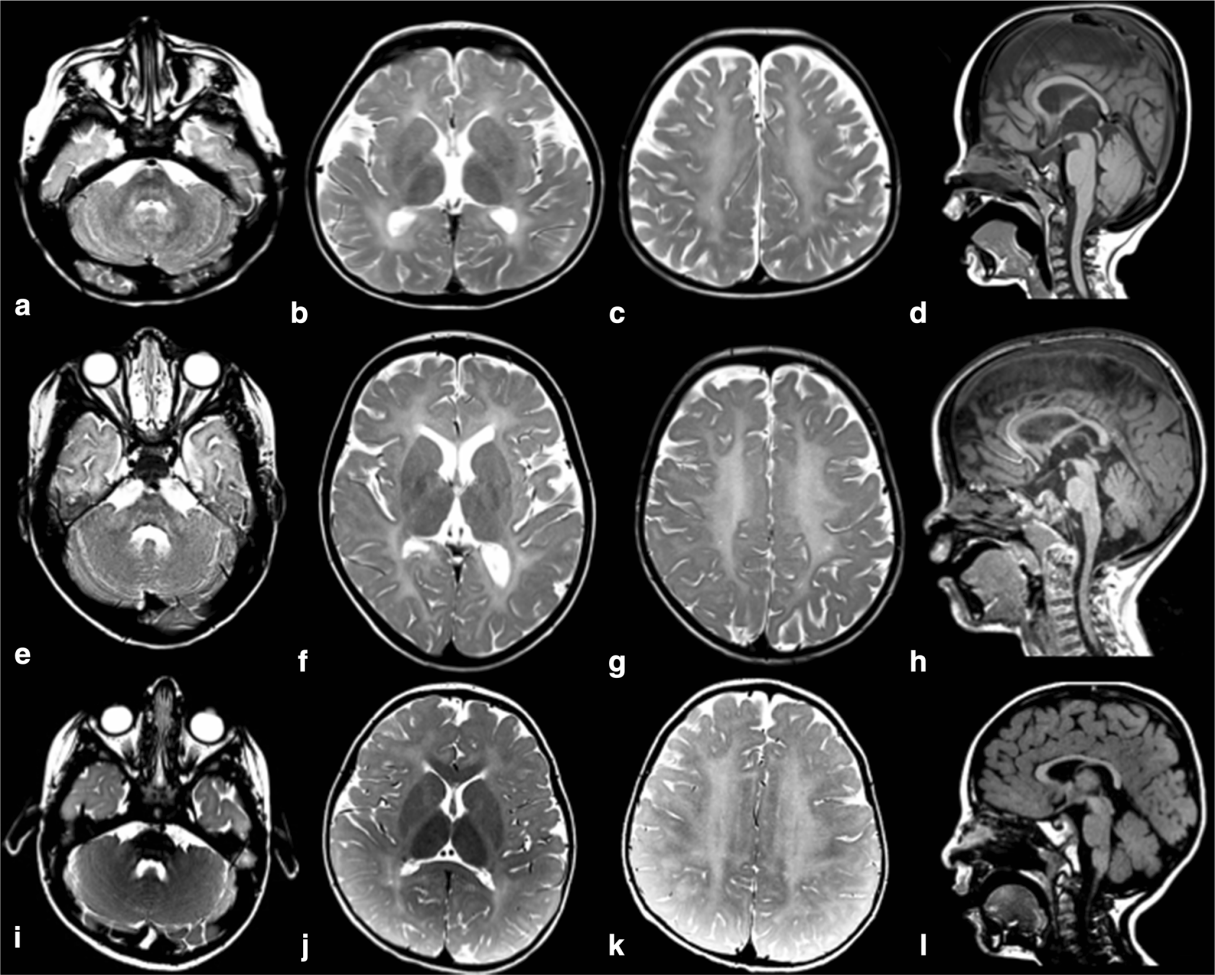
MRI findings in H-SMD. Axial T2-weighted images (a–c, e–g, i–k) and sagittal T1-weighted images (d, h, l) of patient 7 at age 4 years (first row), patient 8 at age 5 years (second row), and patient 1 at age 4 years (third row). The supratentorial white matter shows a diffusely elevated T2 signal (b, c, f, g, j, k), with unremarkable T1 signal (d, h, l) indicating hypomyelination. Signal of the basal ganglia is normal (b, f, j). The cerebellum has a normal volume (a, d, e, h, i, l). Signal of the superior cerebellar peduncles is hyperintense in all patients (a, e, i). Patient 7 has also signal elevation of the hilus of the dentate nucleus (a). Patient 8 of the external part of the middle cerebellar peduncle (e). Patient 1 has relatively better myelination of the brainstem, corpus callosum and deep gray nuclei
